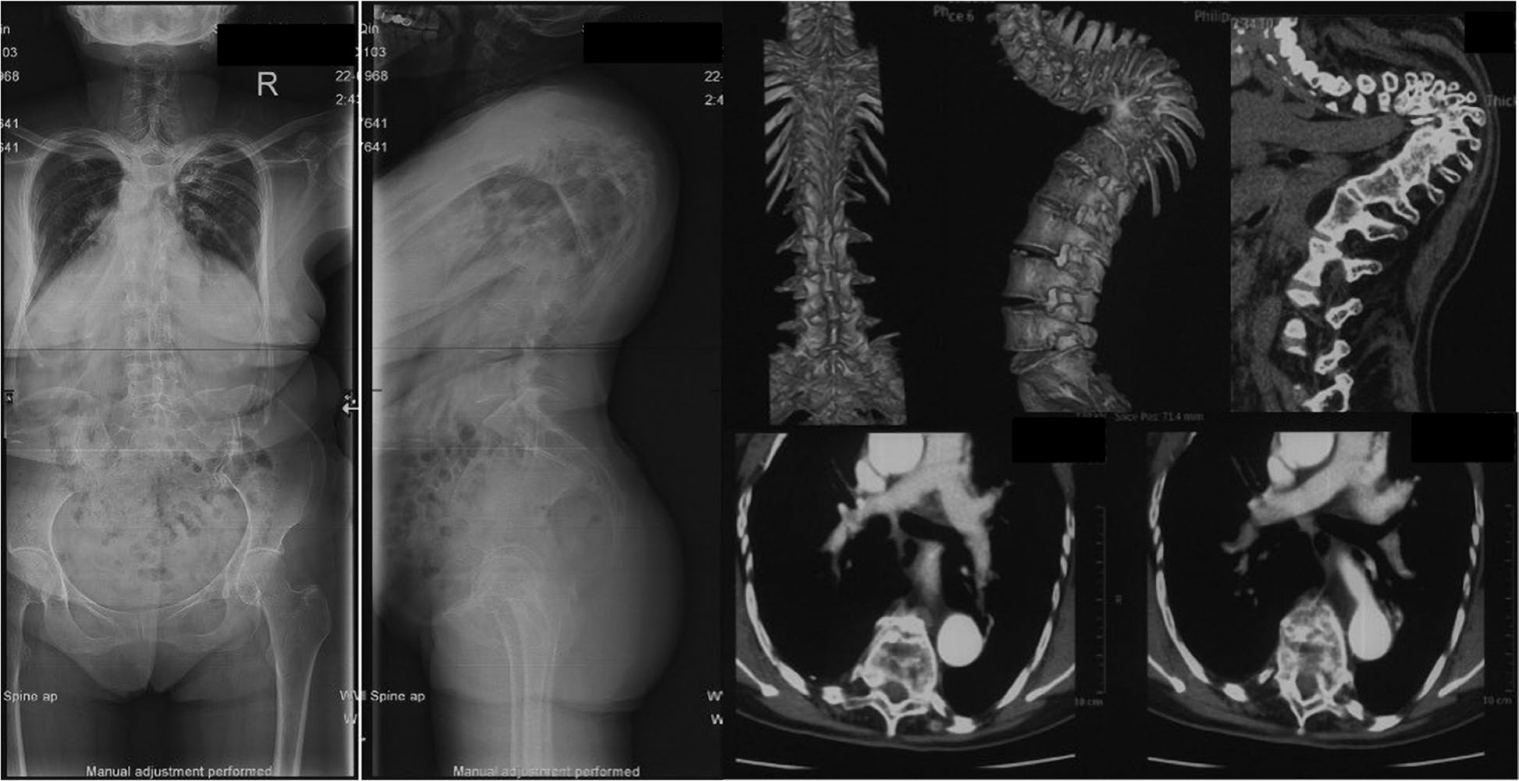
A 59 years old patient presented with weakness and numbness of left lower limb. The kyphotic angle of T2-6 was 111.1° and SVA was measured -2.2 cm. CT scans showed that the aorta was located laterally to the costal head and the costal neck at apex vertebral level (Grade 3)
